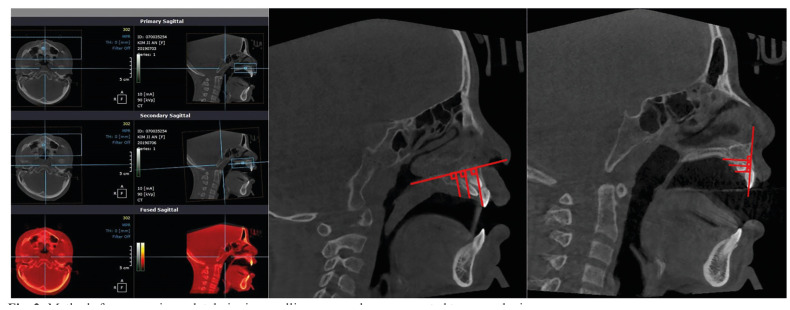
Methods for comparing palatal gingiva swelling on cone beam computed tomography images.
